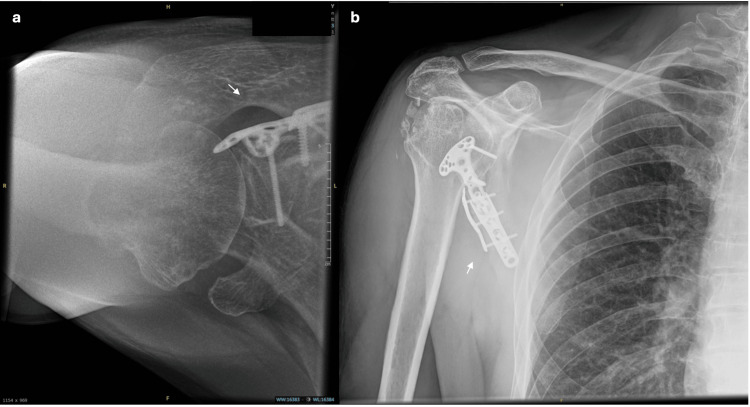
Three-month post-operative radiograph. a. Axial shoulder X-ray. b. Anterior-posterior shoulder X-ray. Figures 10a-10b show evidence of a good fracture union three months post-operatively (white arrows).
